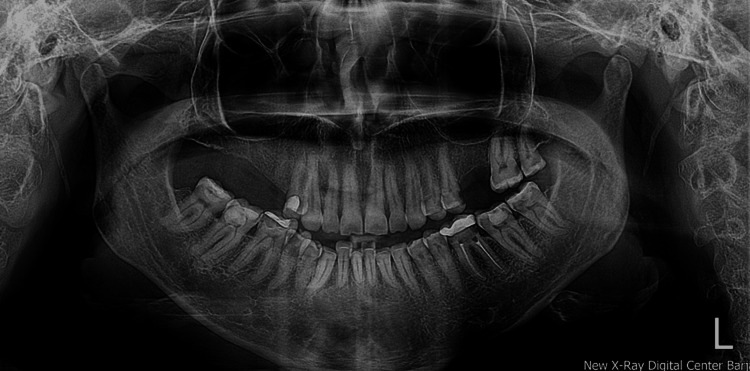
Digital panoramic radiograph of the patient
